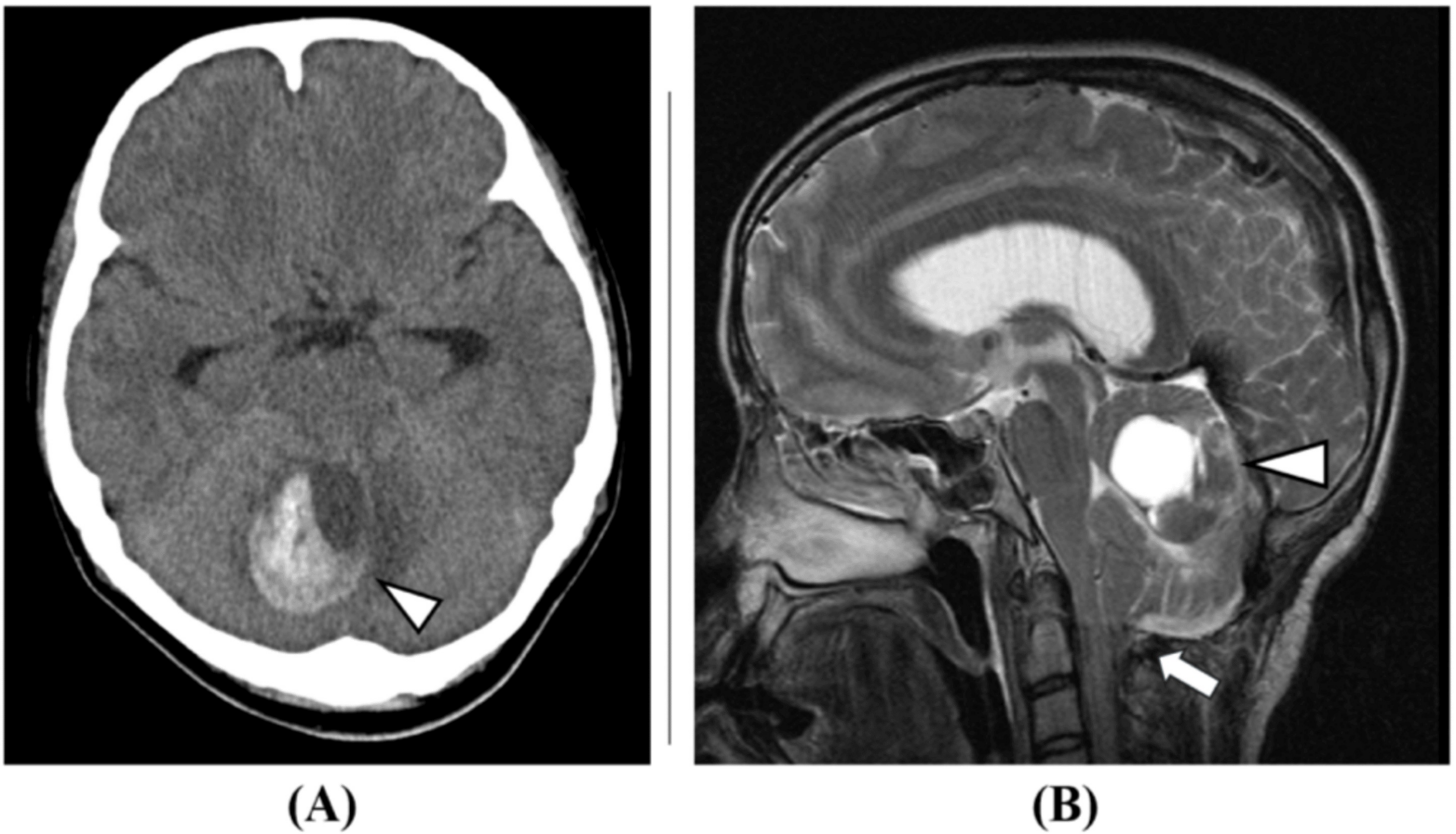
Brain imaging studies (one year and five months after foramen magnum decompression): (A) brain CT image (axial view) and (B) brain MRI image (T2 sagittal). A cerebellar hemorrhage with a maximum diameter of 42 mm was observed (arrowheads in A and B). The cerebellar tonsil herniation has improved (arrow in B).
